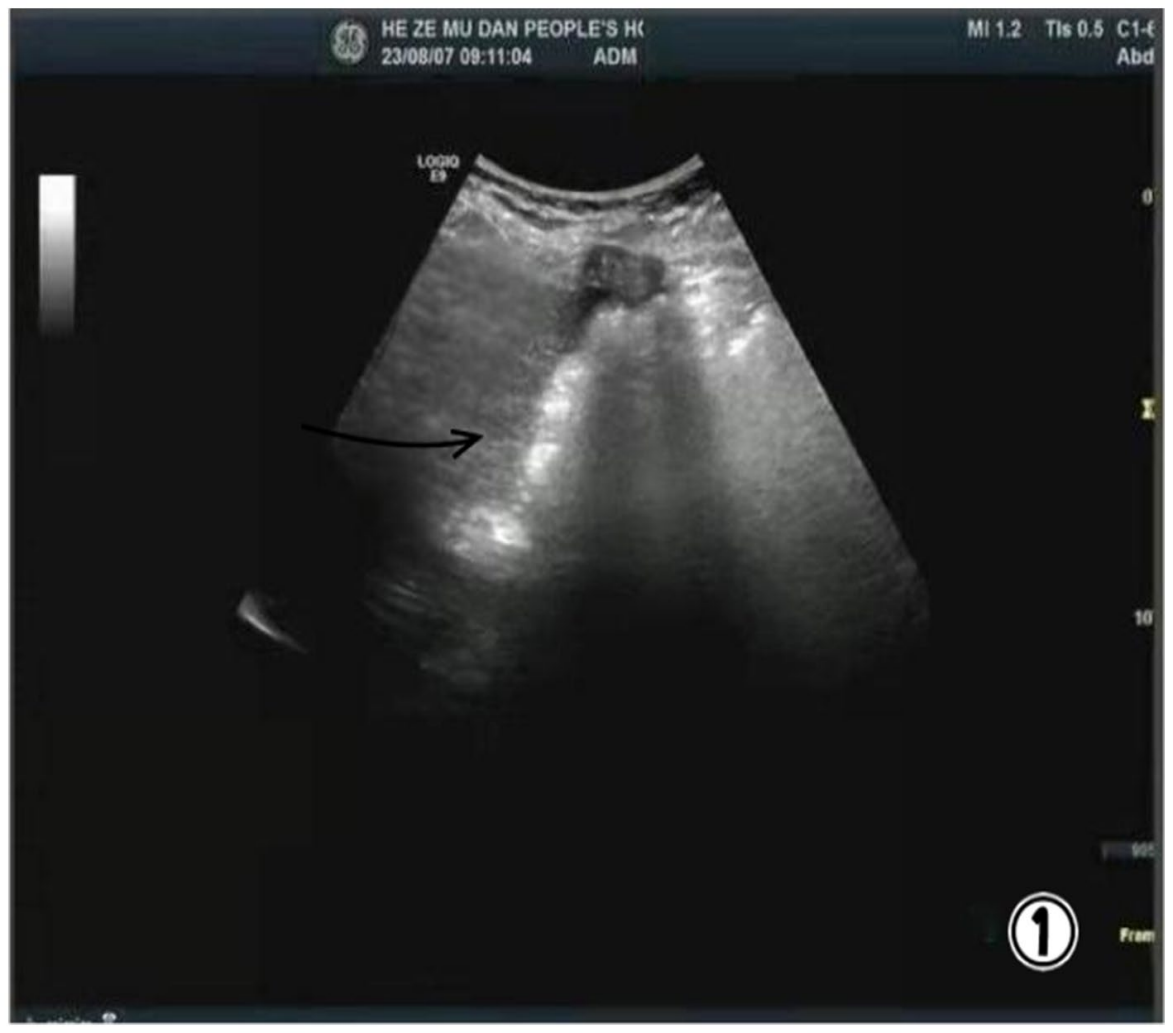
Ultrasound image showing gallstones.
